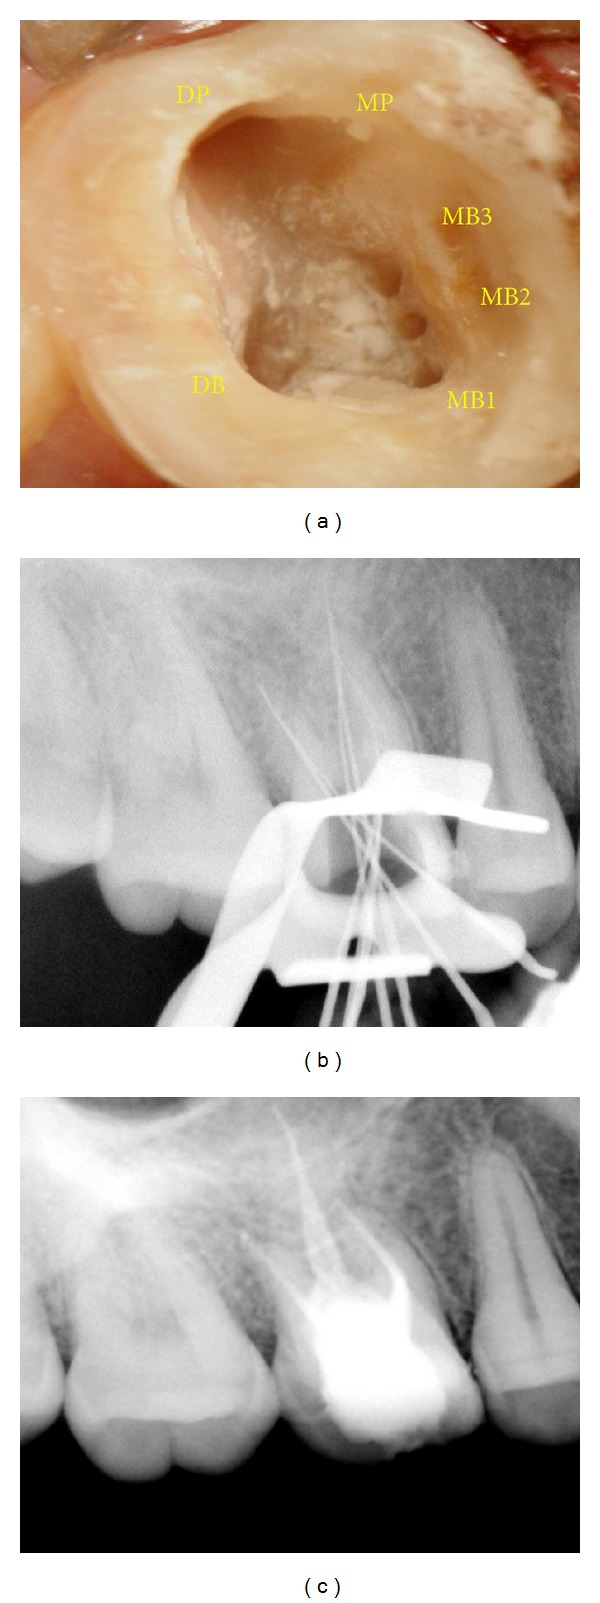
(a) Access opening showing three MB, two palatal, and one oblong distobuccal canal orifices. (b) Working length radiograph. (c) Postobturation radiograph.
